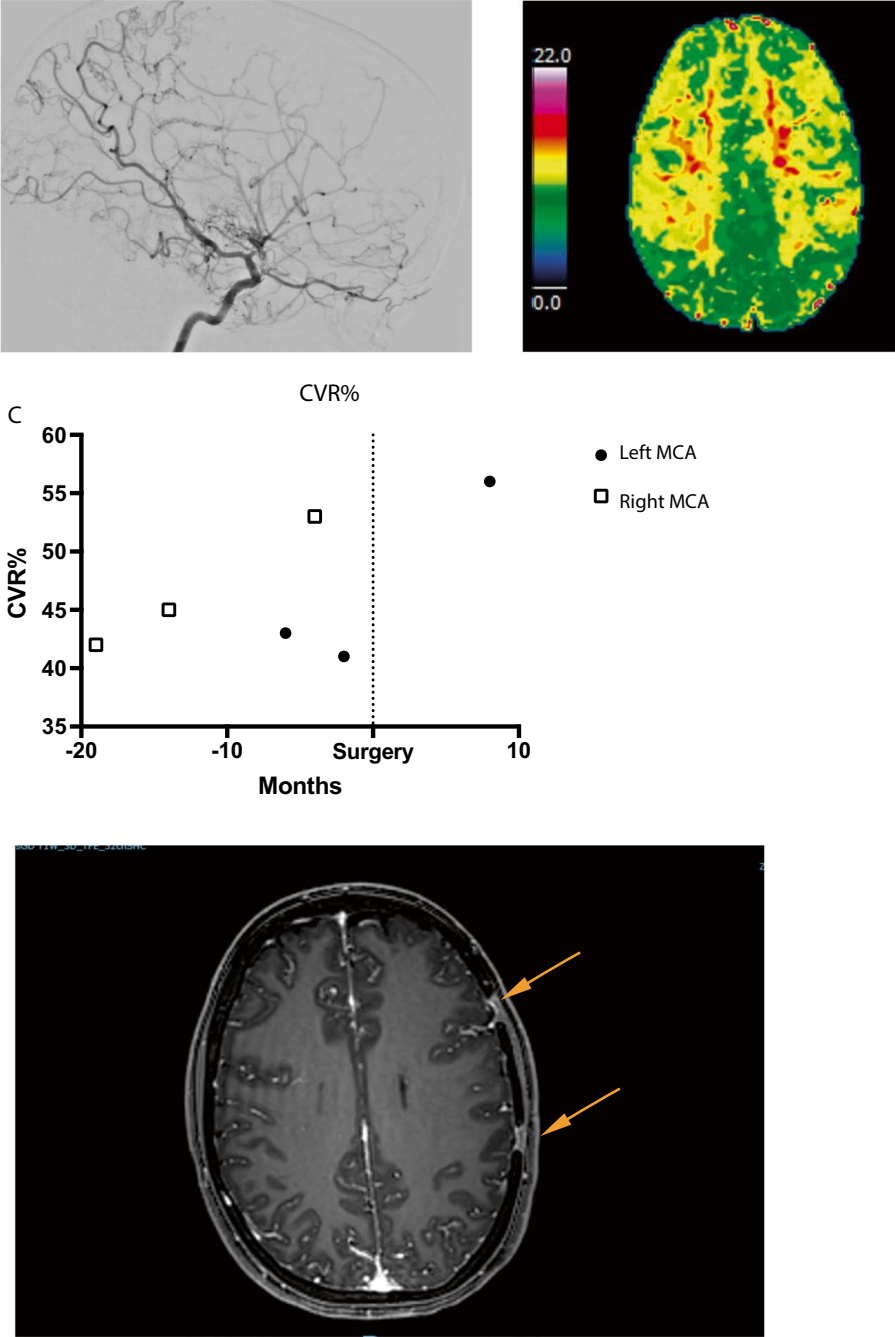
Patient 6, a 26-year-old female with bilateral MMD. A DSA of right ICA. B TTP map showing delayed TTP bilaterally especially in the MCA territory. C CVR on the left side decreased from 43 to 41% pre-op. We started with a left-sided MBH. Post-op MRI showed improved CVR to 56%, and even on the right side, the CVR improved to 53%. Values are presented in relation to when surgery was performed, on each side separately. However, due to symptoms and a strong patient wish, surgery was also performed on the right side. D Ingrowth of contrast enhancing blood vessels through burr holes after left-sided MBH
